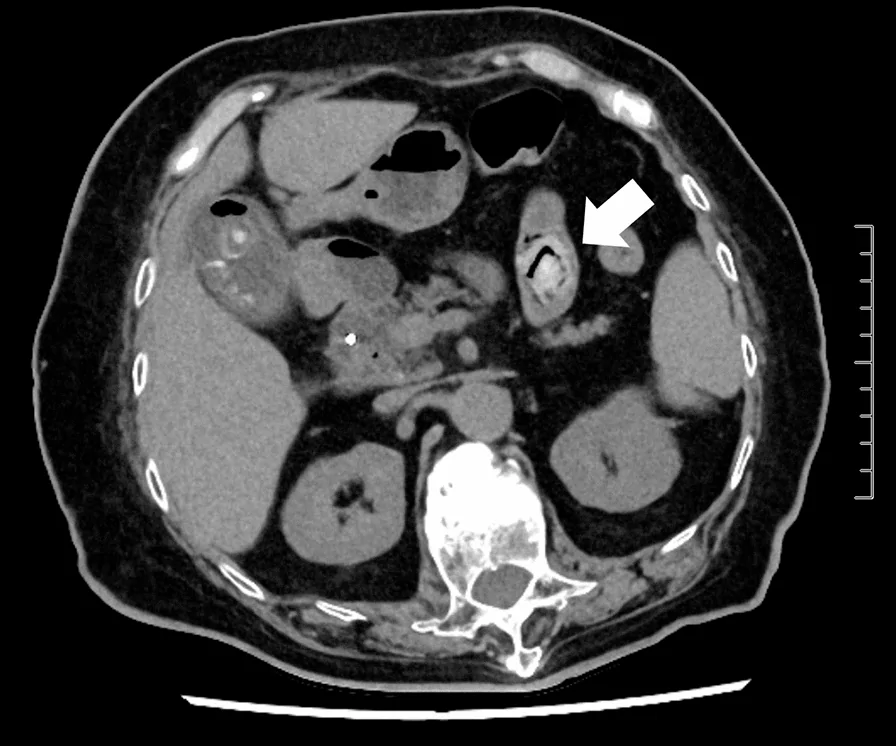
Abdominal CT scan at the second visit BEFORE ERCP revealing foreign body in the upper jejunum (arrow)
